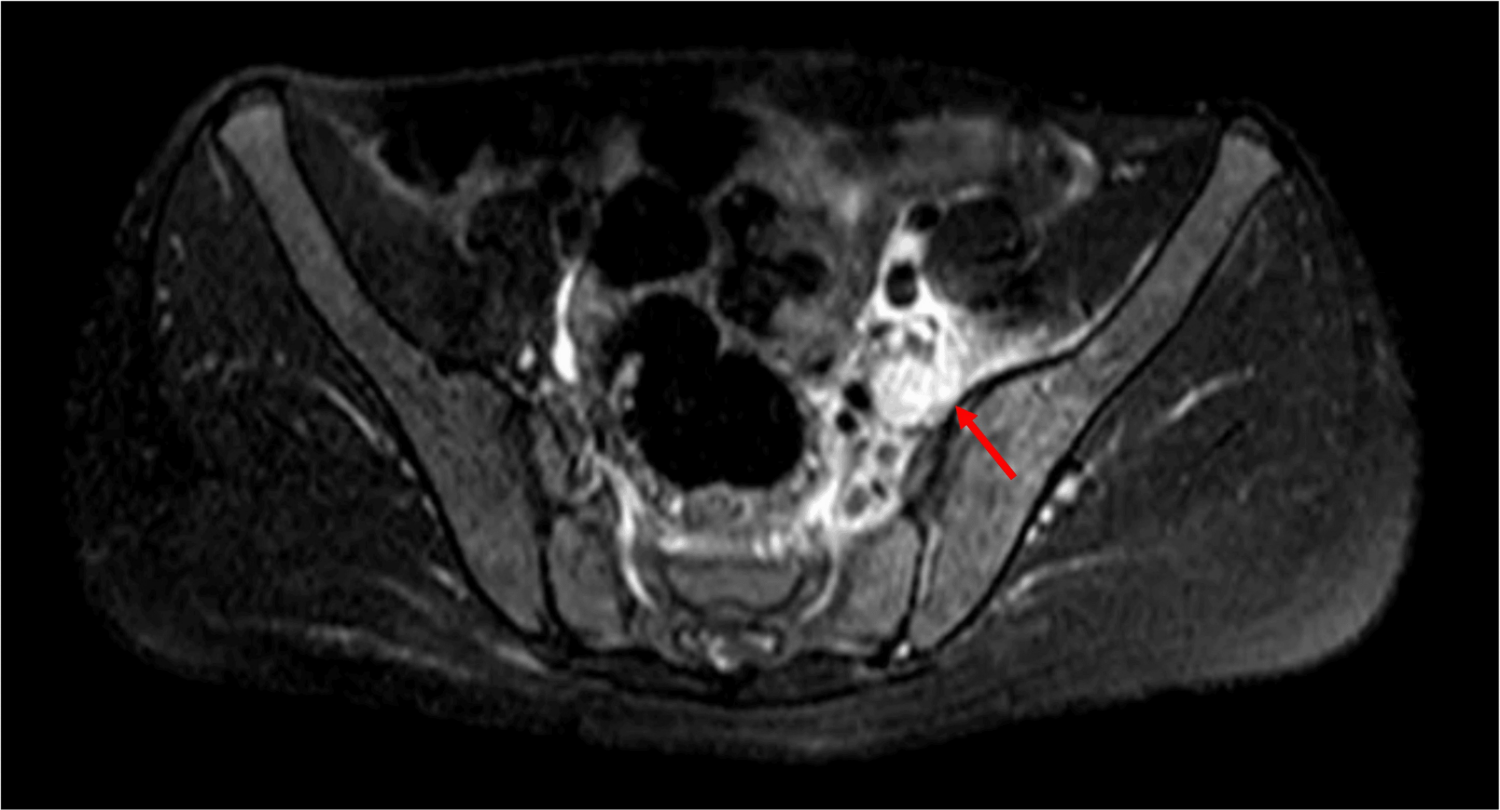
Pelvic MRI As seen in the image, marked by the red arrow, there is moderate edema of the soft tissues located between the left iliopsoas muscle, the iliac bone, and the lumbar spine, accompanied by moderate edema of the iliacus muscle.
